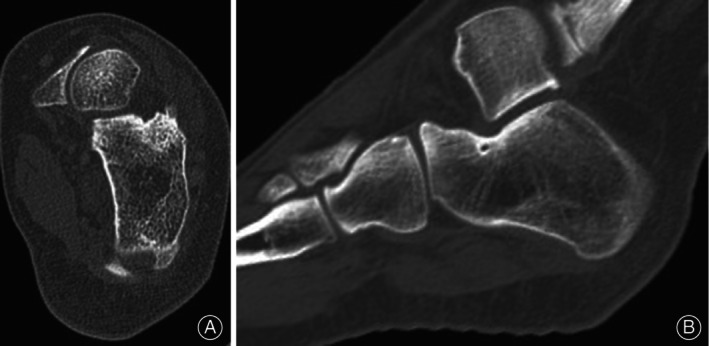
Post‐operative CT images of osteoid osteoma in the calcaneus in an 11‐year‐old male. Post‐operative axial (A) and sagittal (B) images of CT at 12th month showed that the nidus shrank and achieved significant bone remolding.
